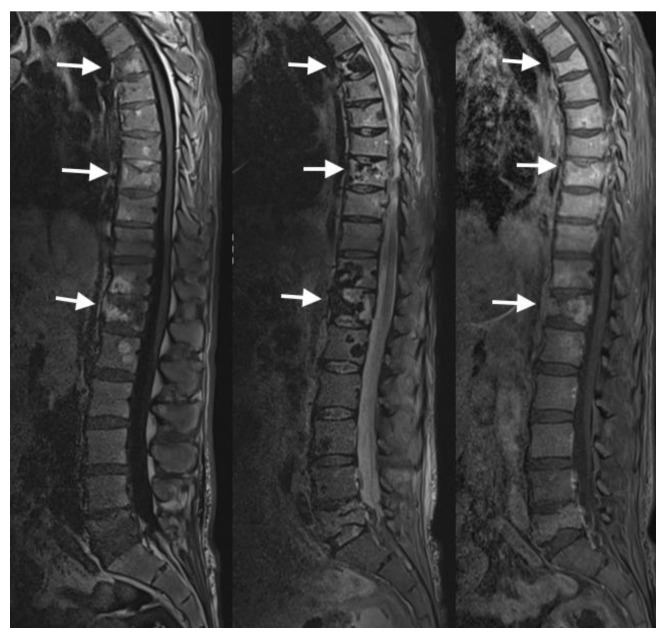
In the sagittal MRI sequences of T1, STIR, and post-contrast T1 from left to right, the arrows show multiple marrow replacing lesions throughout the osseous structures consistent with extensive metastatic disease in this melanoma patient. Multilevel chronic pathological compression fractures within the spine without significant retropulsion or spinal cord compression.
